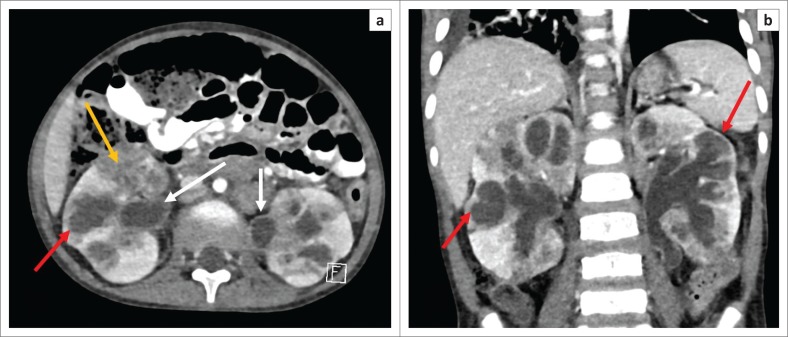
Post-contrast computed tomography (CT) (axial, coronal) shows: (a and b) bilateral hydronephrosis with non-uniform dilatation of the calices with areas of cortical thinning (red arrows). The calices are irregular and there is diffuse urothelial enhancement (white arrow). There are also patchy heterogeneous areas of reduced enhancement of the renal parenchyma with mass like areas of confluence (yellow arrow). The kidneys are enlarged.
